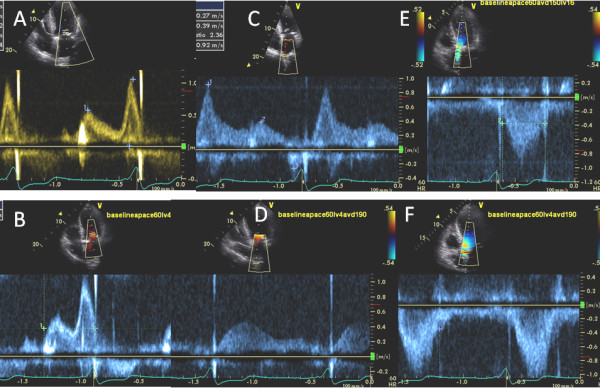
Effect of AV delay optimization on diastolic filling. Mitral inflow (A and B), pulmonary vein inflow (C and D), and LV outflow PW Doppler (E and F) at baseline (Apace 60 AV delay 150, LV 16 ms): A, C, and E) and optimal (A-paced 60 beats/min, AV delay 190 ms, VV0 ms: B, D, and F) pacemaker settings. An increase in A wave duration occurred (B), and pulmonary vein pattern changed so that systolic fraction increased, S wave deceleration time increased (D) and increased pulmonary vein atrial reversal (C) was abolished indicating reduction in left atrial pressure. An improvement in percentage LV ejection time also occurred (F).
